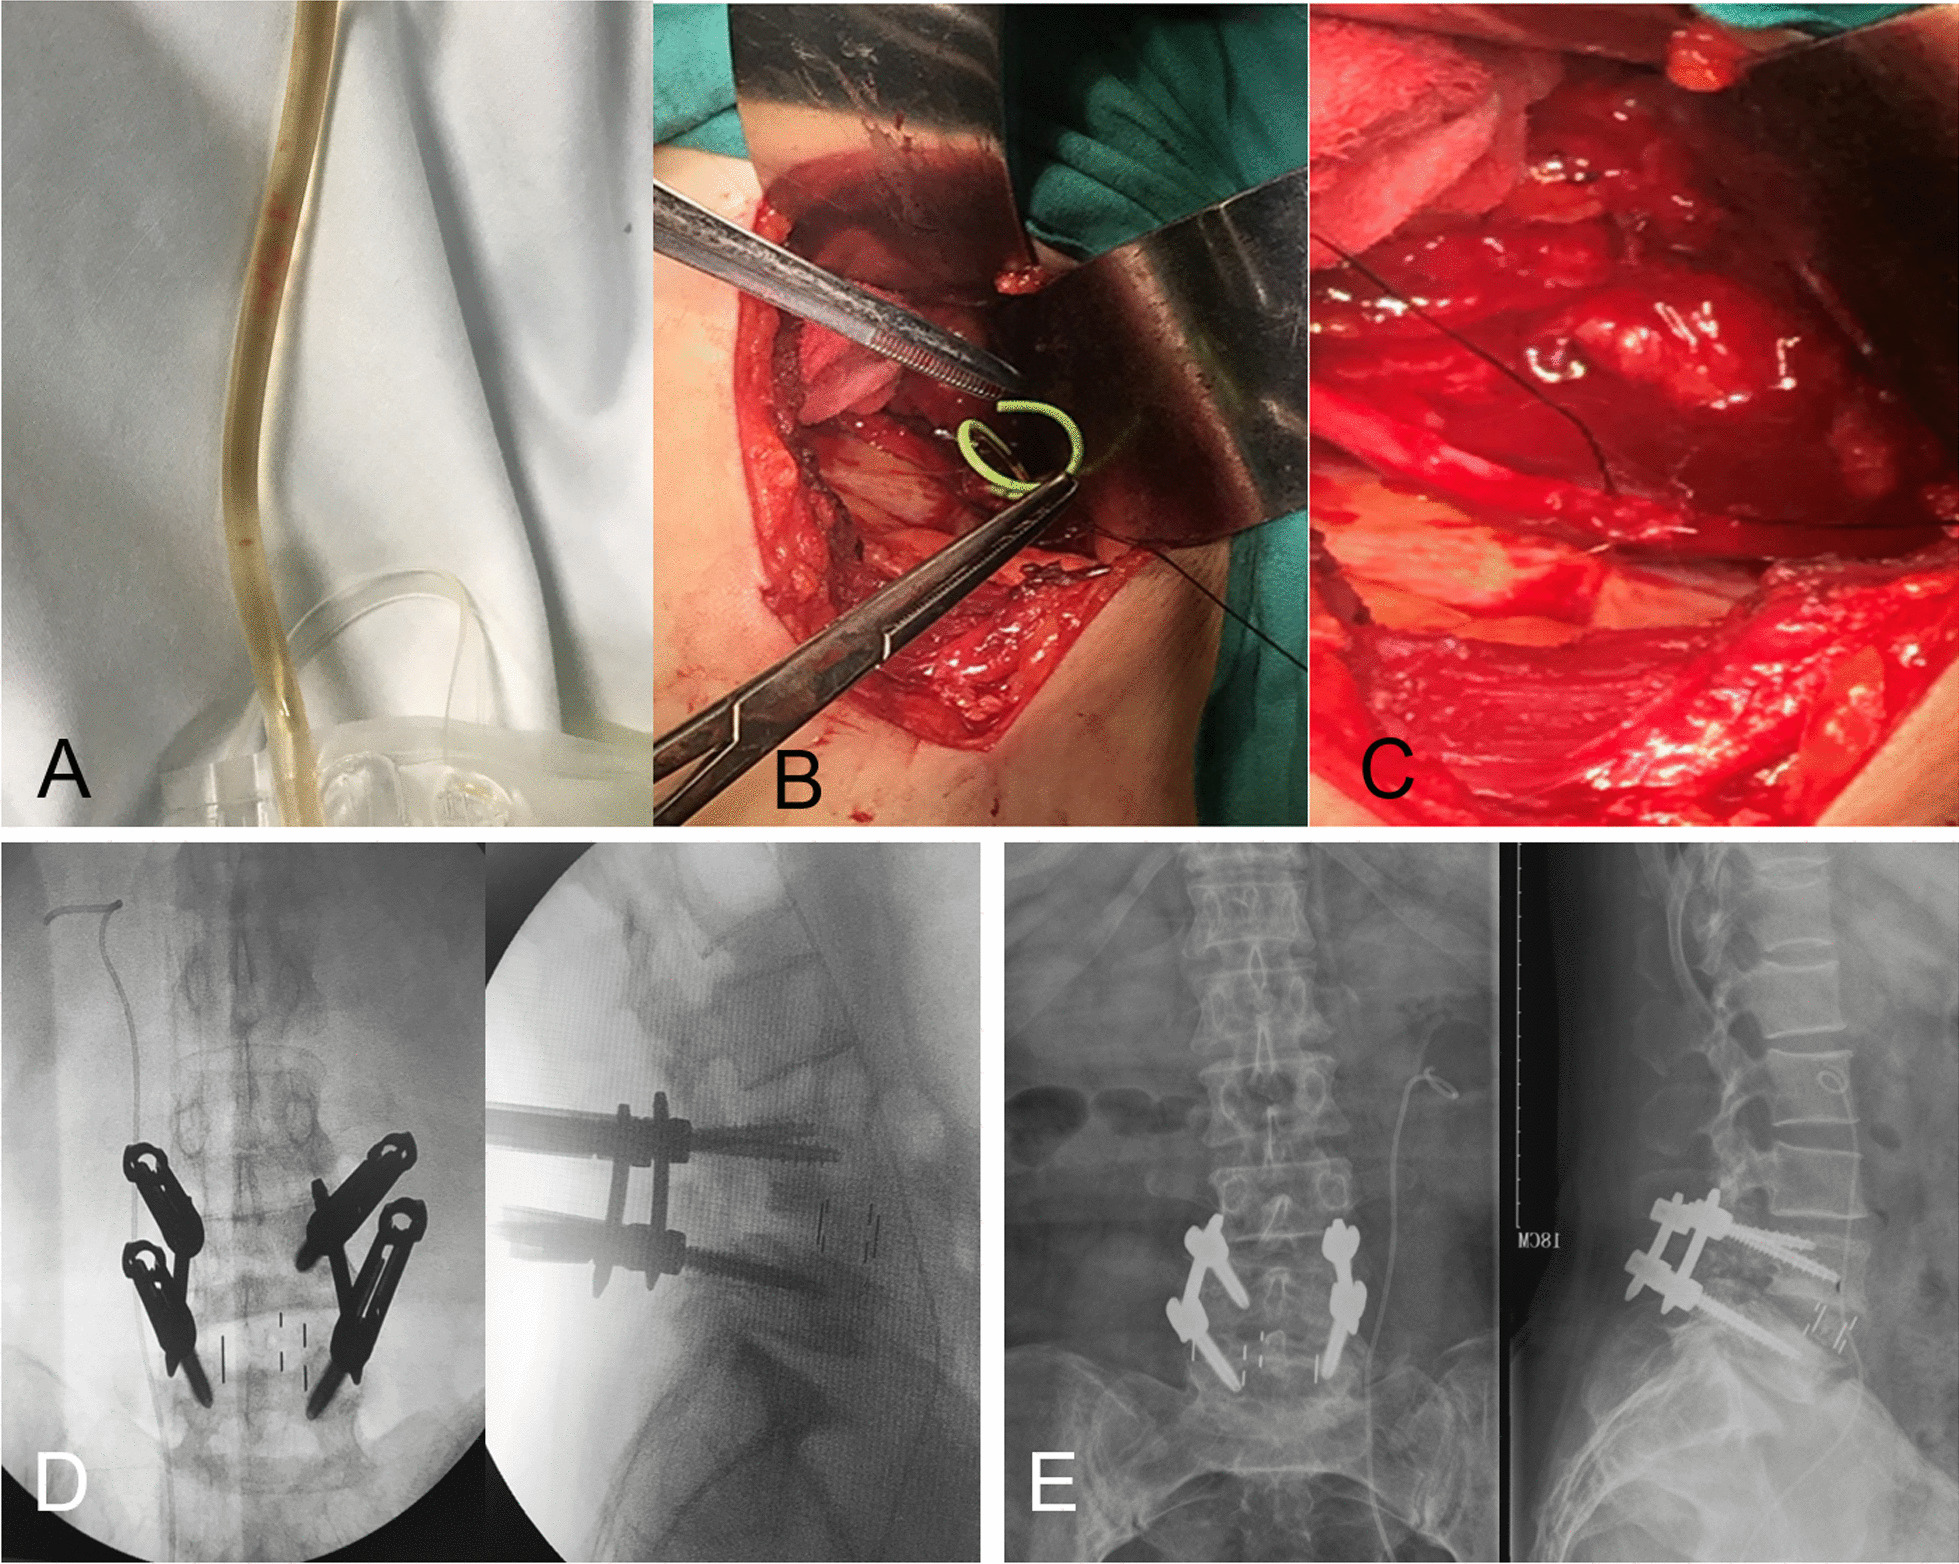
Photos of left ureter repair during surgery and postoperative review imaging. a Showed that hematuria was found in the catheter. b Showed that the ureteral stent was inserted into the left ureter during the operation. c Showed that the left ureter was repaired during surgery. d Showed that the intraoperative X-ray indicated the height recovery of the intervertebral disc and the left ureter double J tube was in good position. e Showed that a good fixation position within 1 year after surgery
